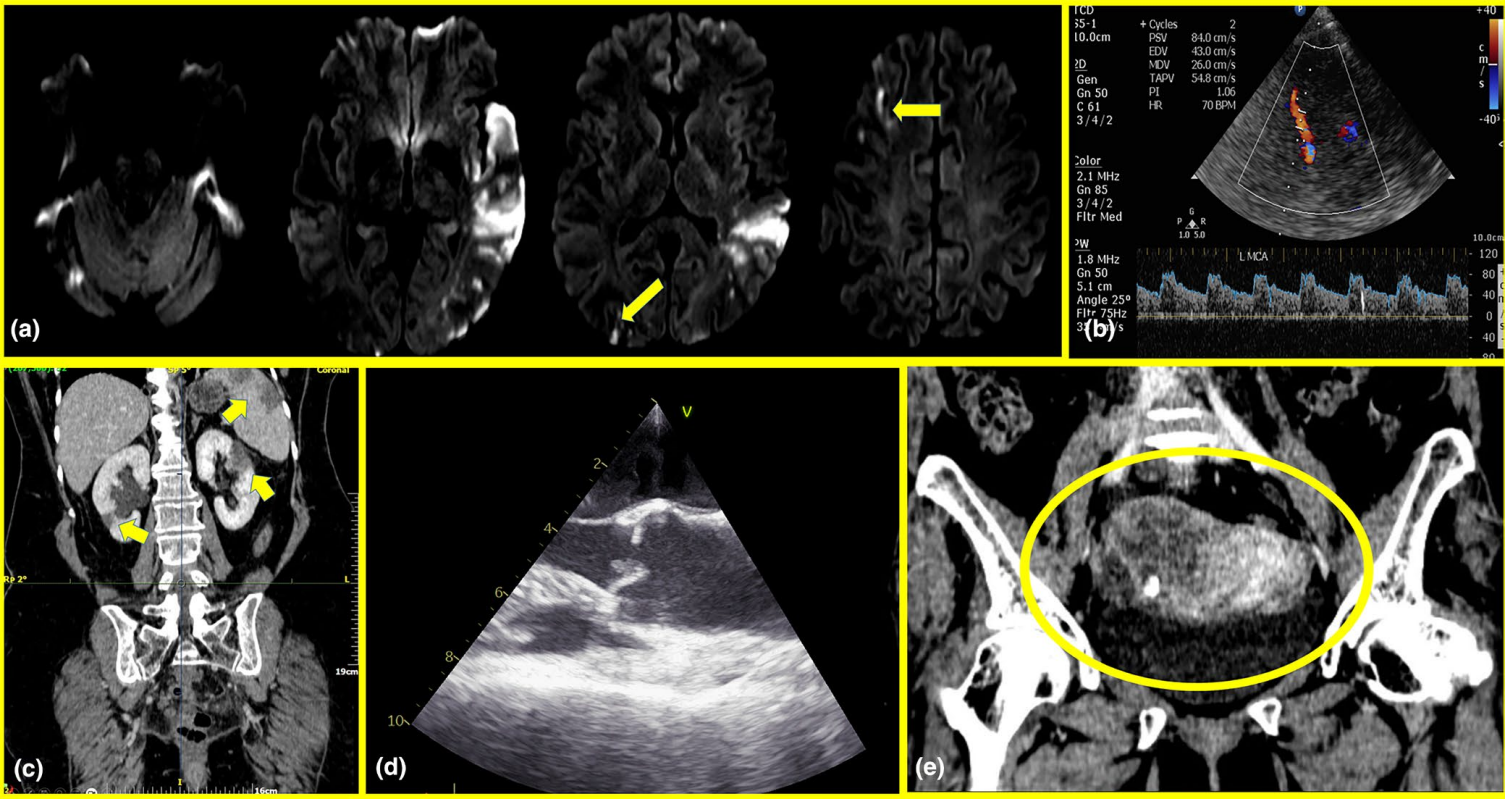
Patient with acute ischemic stroke lesions in multiple arterial territories, as shown in MRI‐Diffusion Weighted Image (a), with positive microembolic detection on the middle cerebral artery as detected by Transcranial Color‐Coded Doppler (b) and multiple visceral infarcts on CT‐scan (kidneys, spleen; (c)), due to non‐bacterial thrombotic endocarditis as detected during transesophageal echocardiogram (d), associated with ovarian mucinous cystadenocarcinoma (CT‐scan; (e)).
